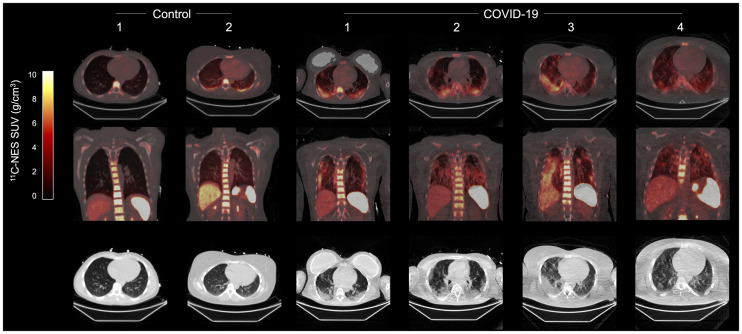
PET/CT images of 4 patients and 2 controls (3 male, 3 female) at 1 h after injection. Upper 2 rows are ^11^C-NES images; lower row is CT images.
